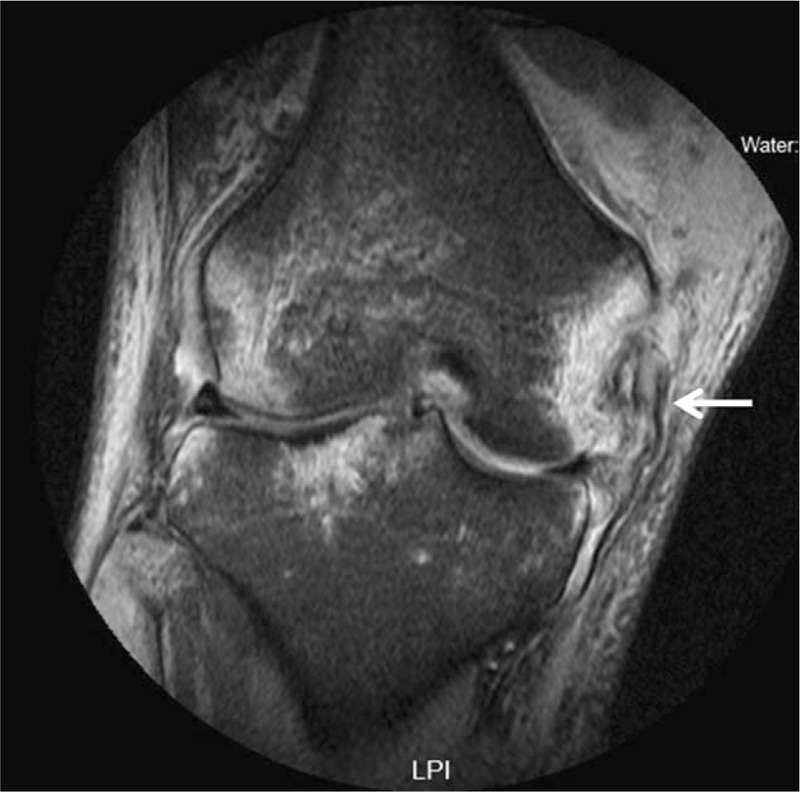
Coronal T2 fat decompression magnetic resonance imaging (MRI). Arrow indicates bony avulsion fracture with intact ligament fibers.
